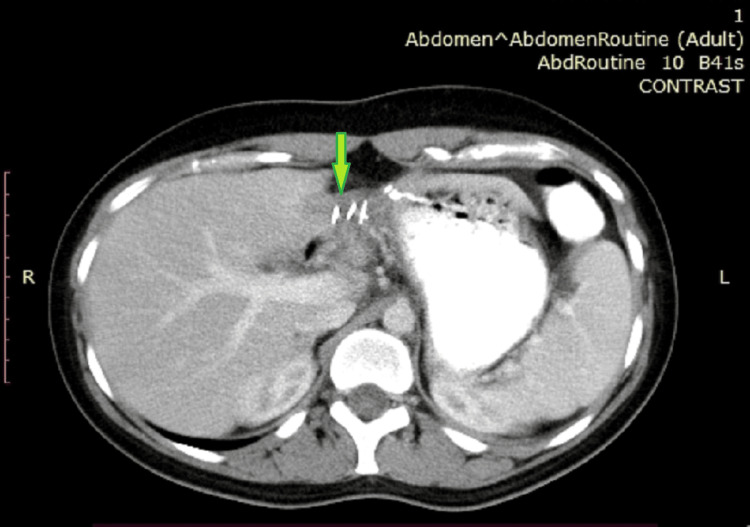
Postoperative CT scan showing no detectable liver pathology. The hepatectomy site is visible on the left and marked by a green arrow. The clips from the GIA stapler are also visible.
